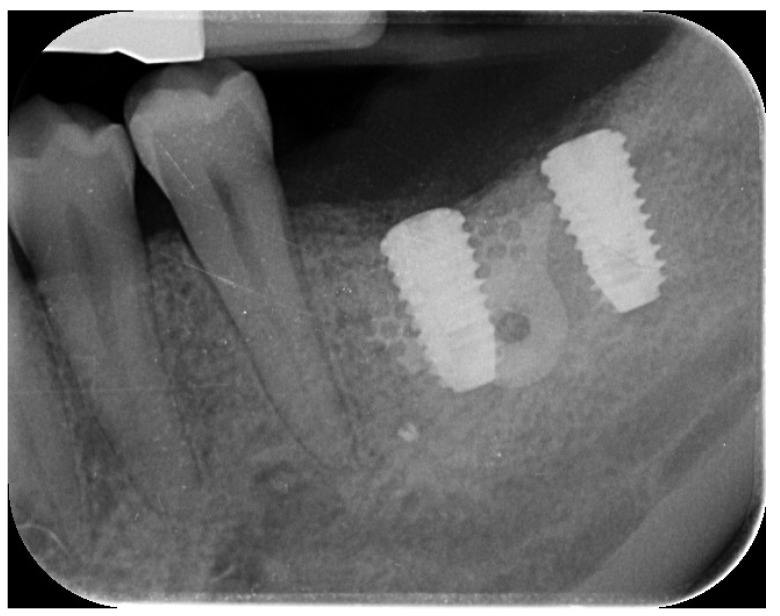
Radiographic assessment showing clear visibility of the buccal portion of the membrane left in situ.
